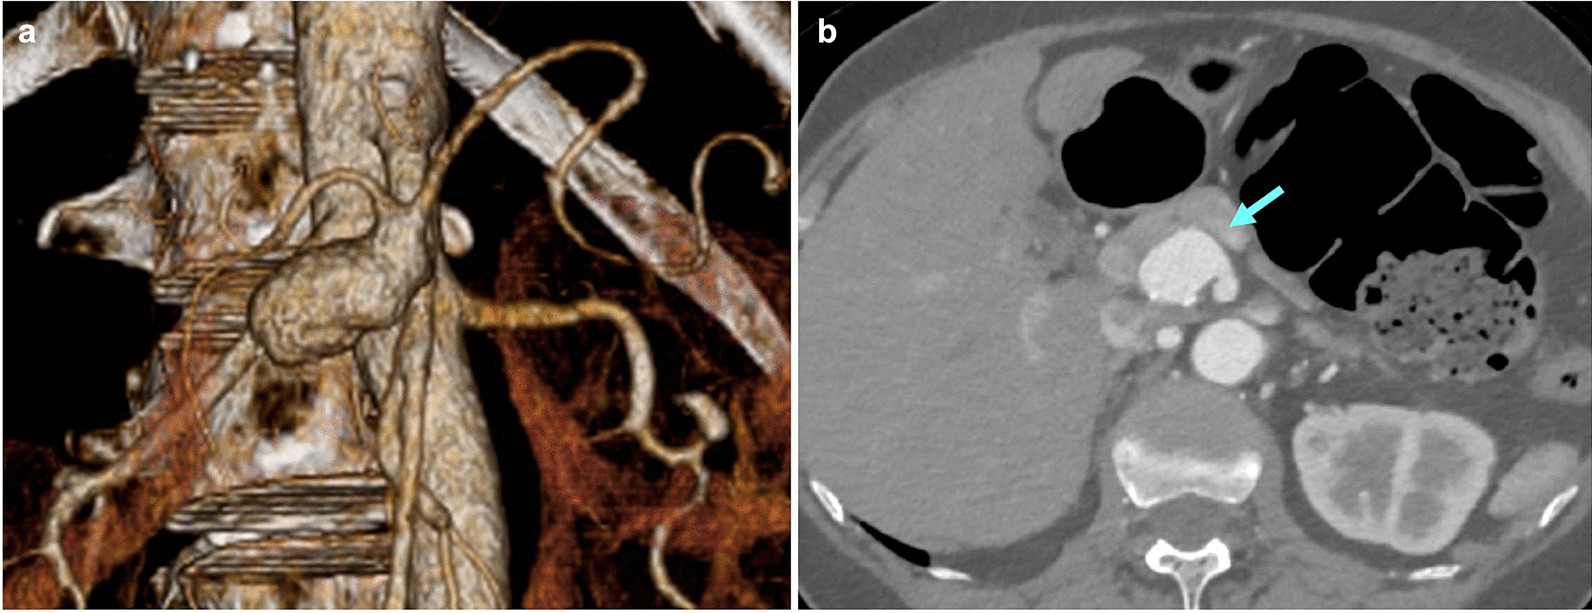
Preoperative enhanced computed tomography findings. A celiac artery aneurysm (shown by the blue arrow) located distal to the celiac artery branched from the celiacomesenteric trunk [panels (a) and (b) showing 3D and axial views, respectively]
